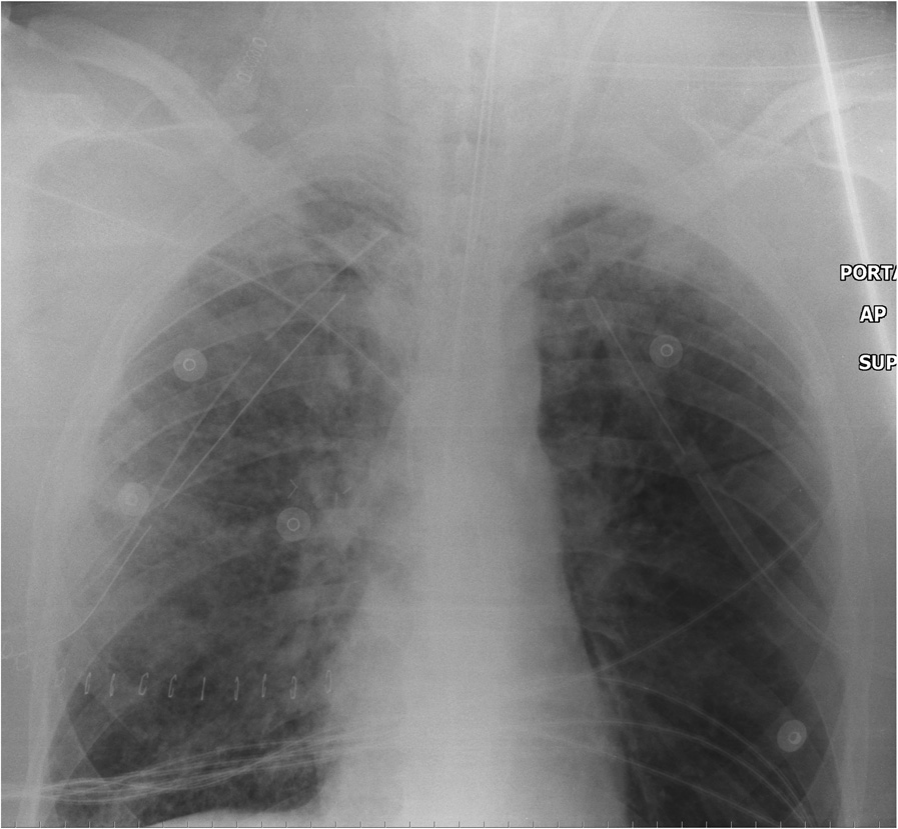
Chest X-ray 48 hours after intensive care unit admission. We see a clear improvement, with decreased bilateral infiltrates, compared with previous X-ray.
